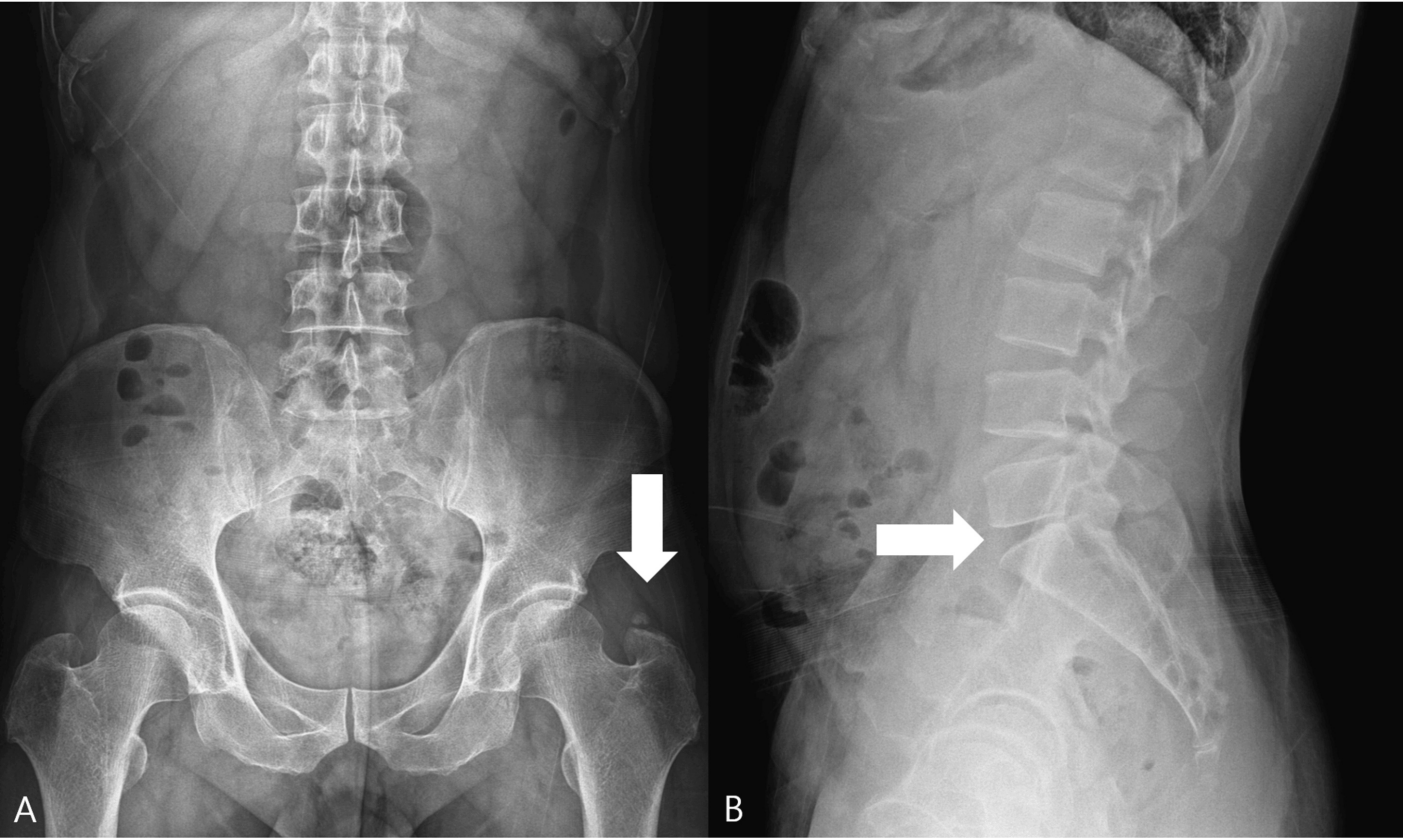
Lumbar spine radiographs. (A) Anteroposterior view showing calcification (arrow) near the left greater trochanter. (B) Lateral view demonstrating L5-S1 disc space narrowing (arrow).
